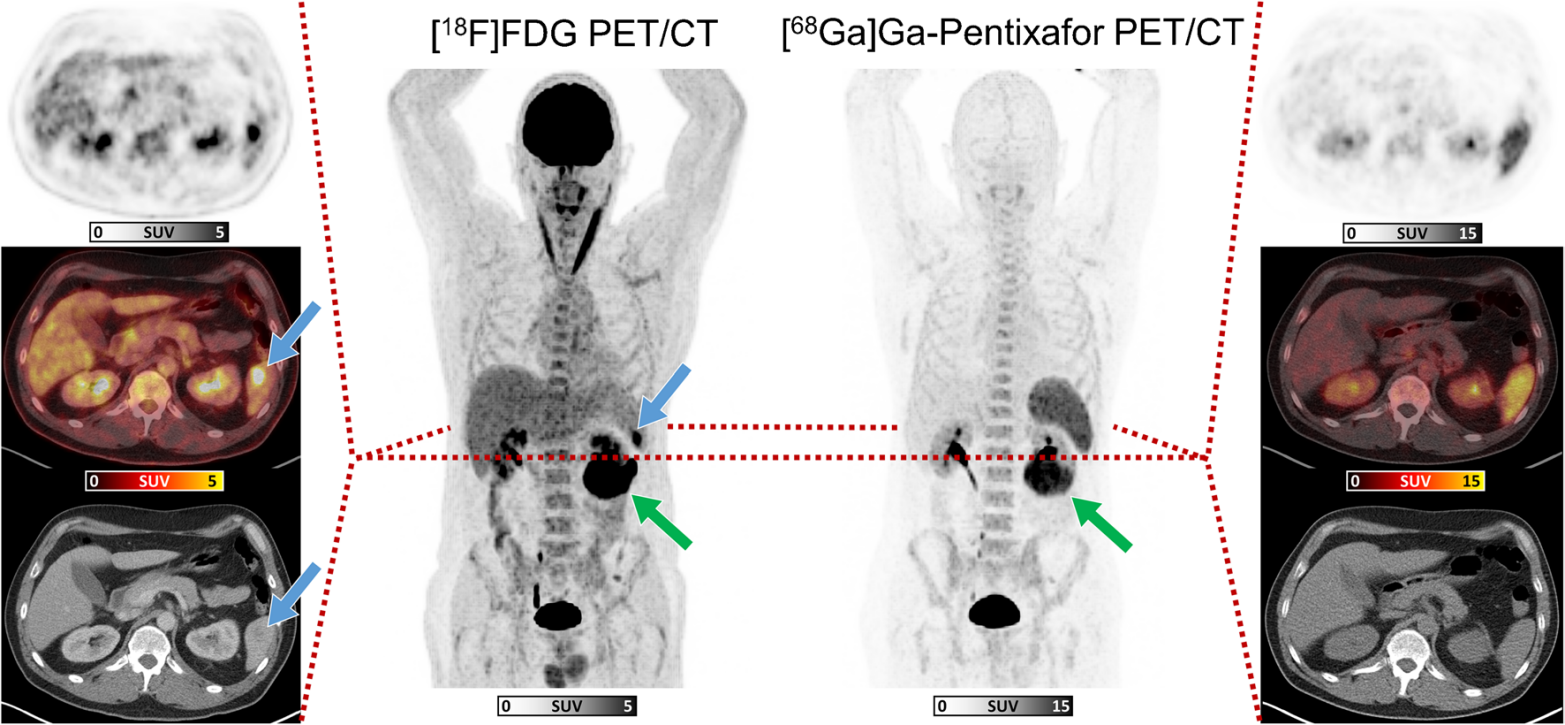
Maximum-intensity-projections (MIP) of [^18^F]FDG PET/CT (center left) and [^68^Ga]Ga-PentixaFor PET/CT (center right) in a 54-year-old male patient affected with extranodal marginal zone lymphoma, with axial sections ([^18^F]FDG: left column, and [^68^Ga]Ga-PentixaFor: right column) of PET (top row), fused image (middle row), and CT (lower row). Blue arrows indicate a splenic lesion, evident only on [^18^F]FDG PET/CT. Green arrows on MIPs indicate a renal lesion seen on both PET scans (not shown on axial sections)
